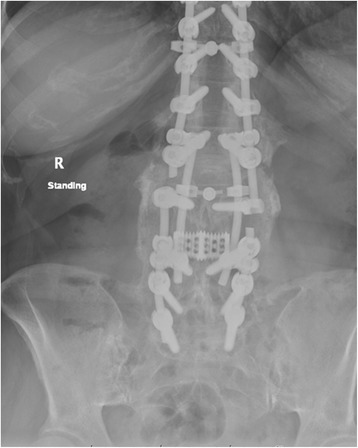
AP postoperative X-rays demonstrating rod repair
